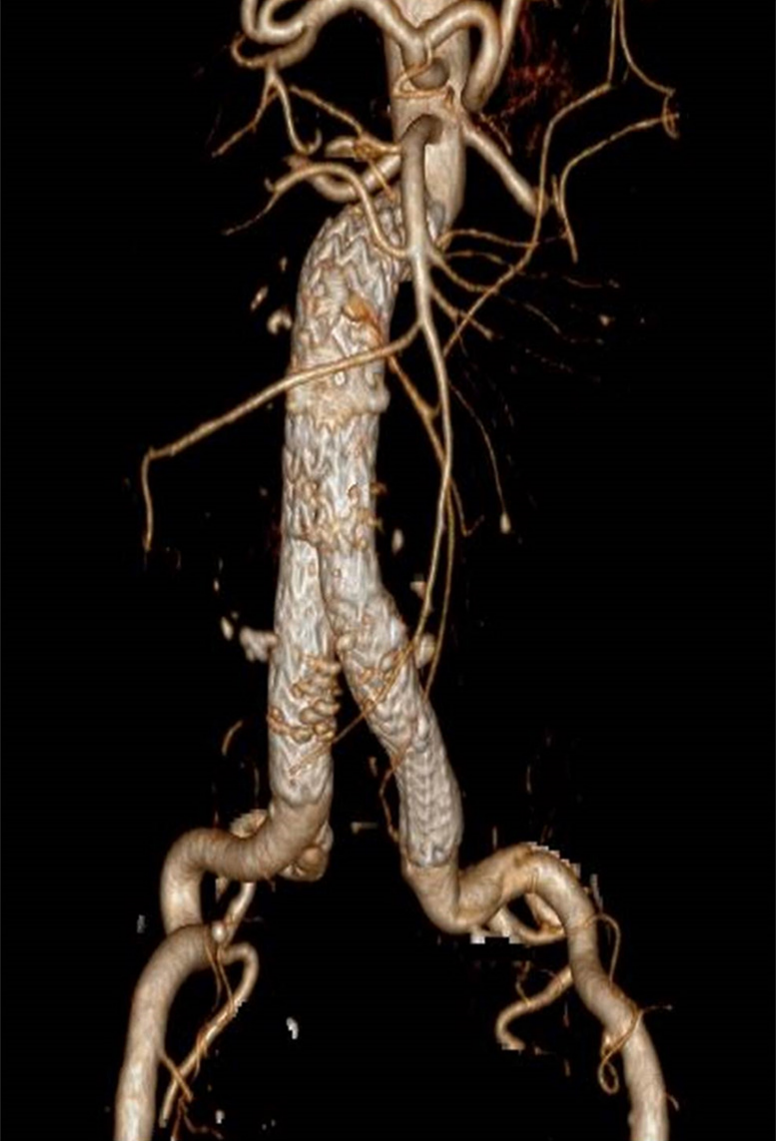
Reconstructed CT angiography demonstrating satisfactory position of the modified stent graft with exclusion of the abdominal aortic aneurysm.
